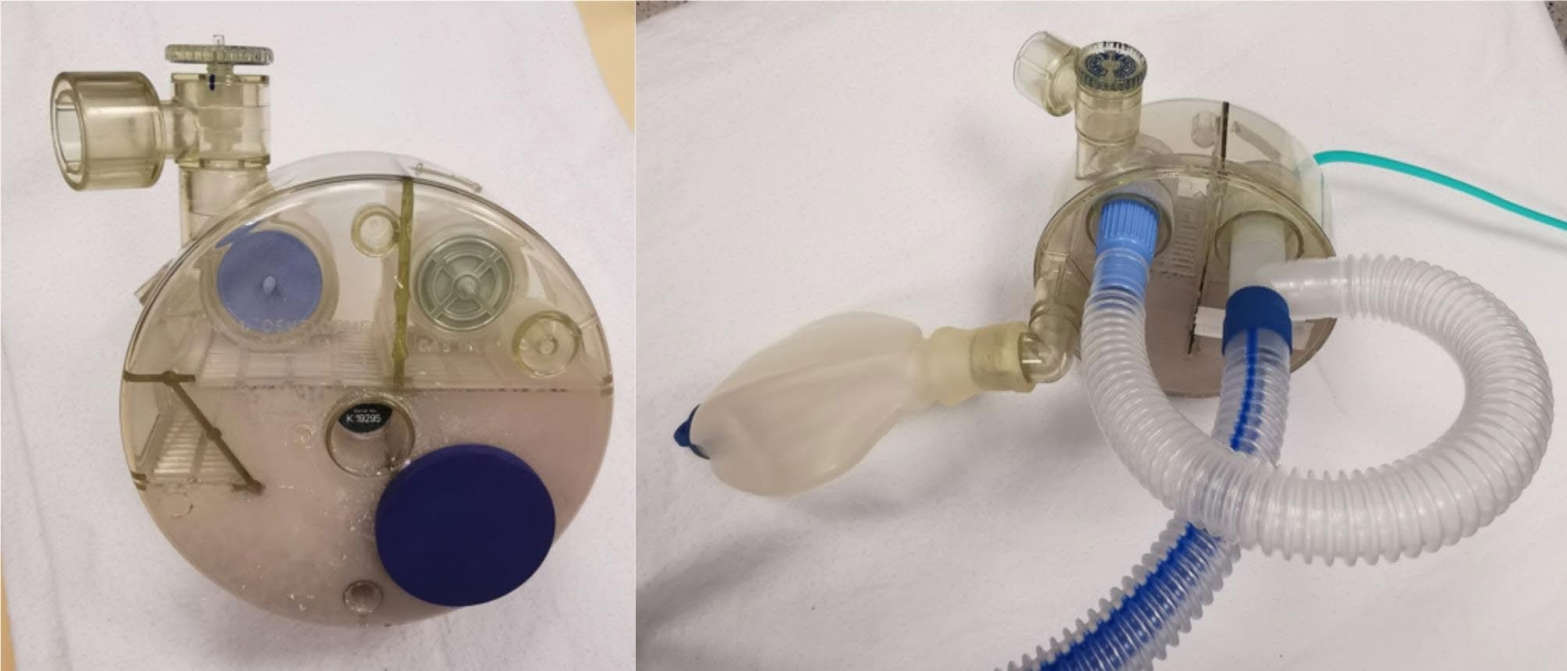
The KAB™ CO_2_ absorber device used to maintain anesthesia during magnetic resonance imaging (MRI). It absorbs CO_2_ in the closed or semi-closed breathing circuit. The green pipe brings fresh gas and permits it to inflate silicon balloons. On the top of the device, there is an APL valve. All are made of plastic (MRI-compatible material)
